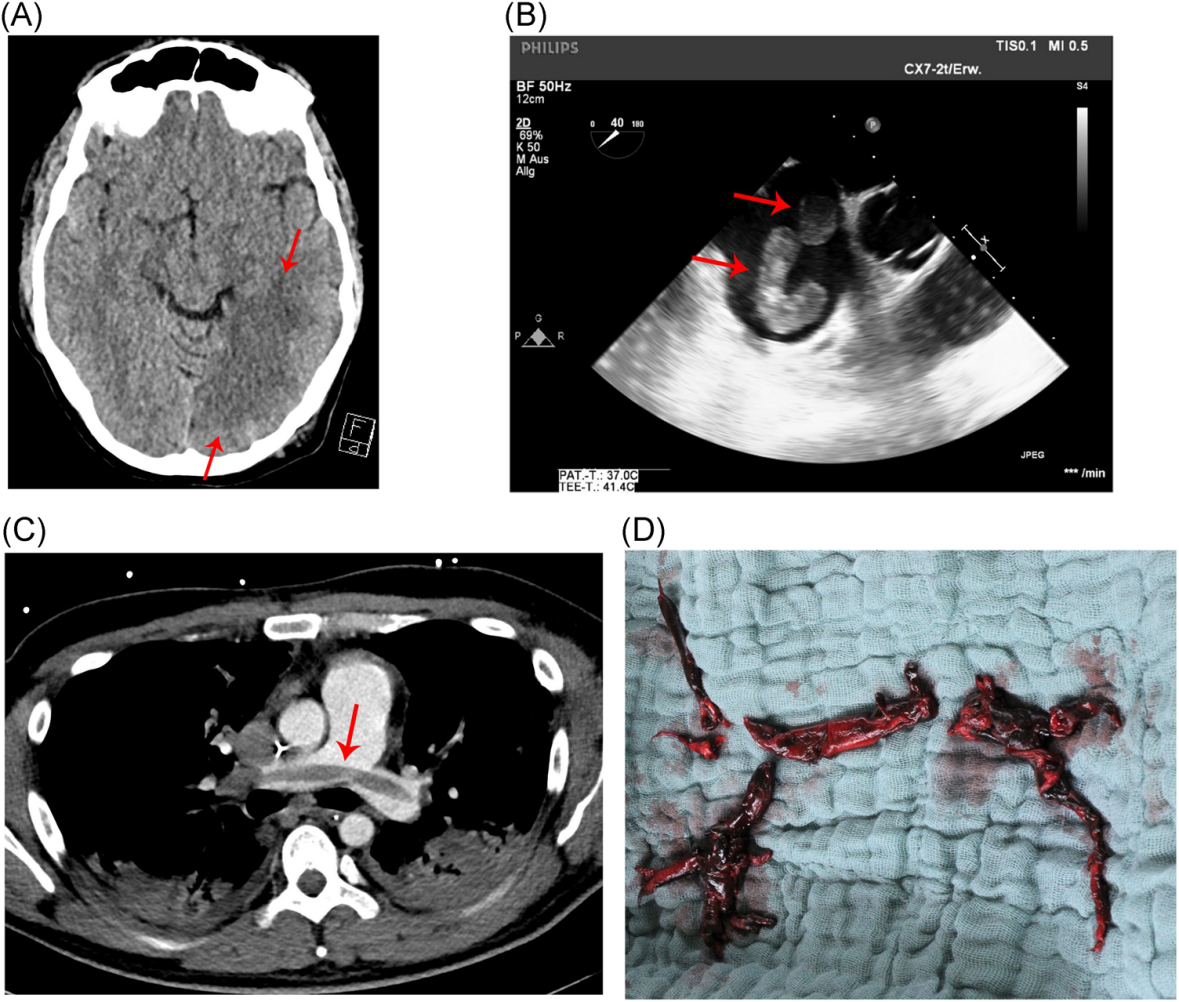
A, Contrast‐enhanced computed tomography (CT) scan of brain demonstrating left‐sided ischemic stroke. B, CT scan of chest demonstrating a large pulmonary embolus extending into the main pulmonary arteries. C, Trans‐esophageal echocardiogram demonstrating thrombus formation (red arrows) within the right ventricle. D, Multiple thrombi retrieved from pulmonary artery intra‐operatively
